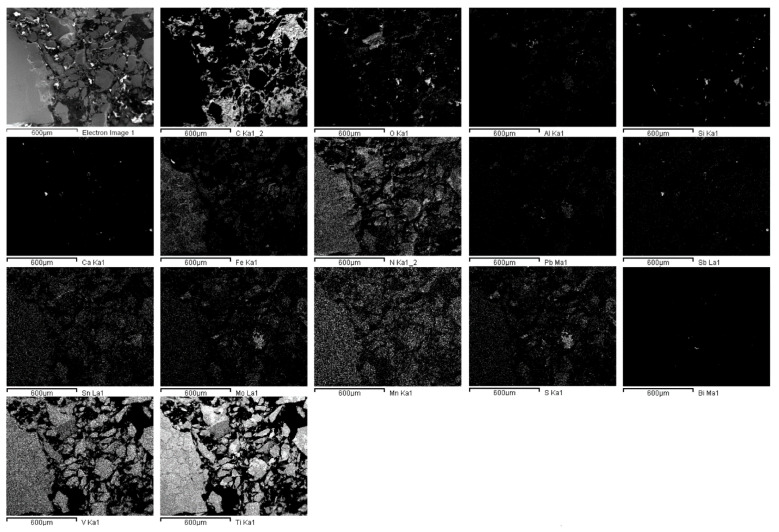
SEM image and the corresponding X-ray elemental mappings showing the distribution of elements in the FeTi-cored wire (C, O, Al, Si, Ca, Fe, N, Pb, Sb, Sn, Mo, Mn, S, Bi, V, Ti).
